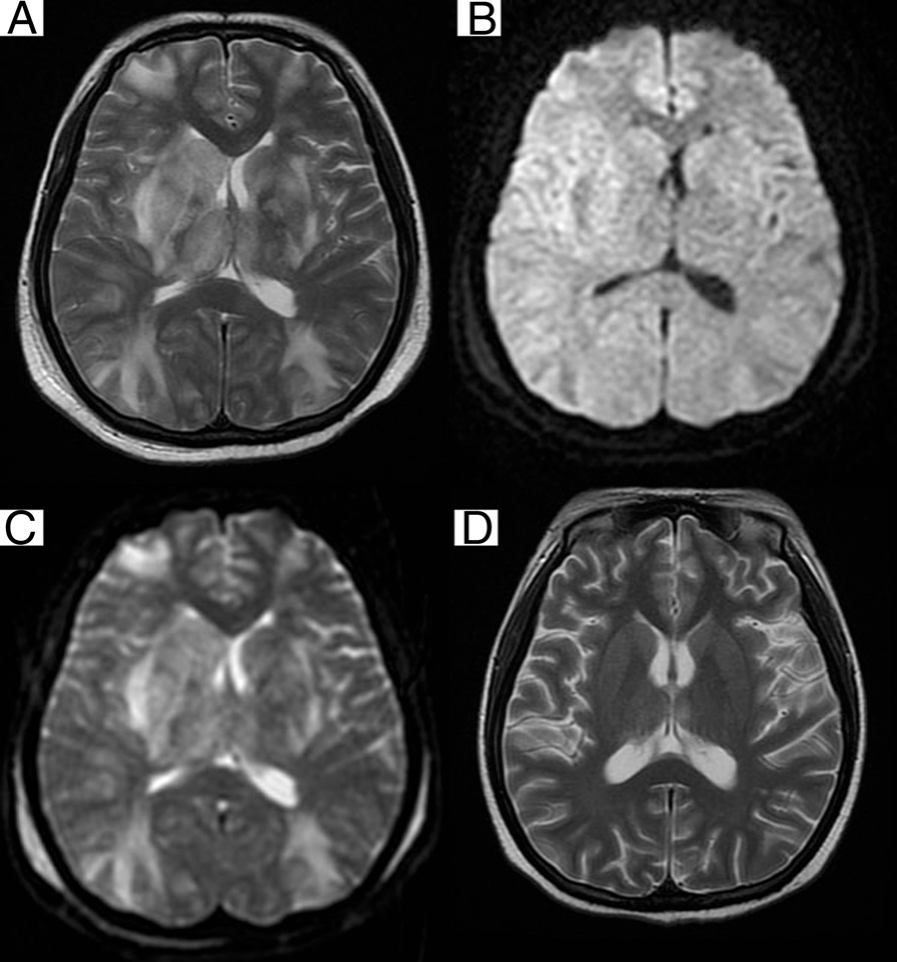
MRI of a 24 year-old SLE patient who presented with seizure. T2-weighted image (A) shows high signal lesions in deep and subcortical white matter bilaterally frontal and parietooccipital but predominantly posteriorly. Diffusion-weighted image (B) shows low-signal intensity area in corresponding lesion areas. Corresponding apparent diffusion coefficient (ADC) map (C) reveals high signal intensity area corresponding to low-signal area on diffusion-weighted image. Follow-up T2-weighted image (D) obtained 3 months after (A) shows complete resolution.
